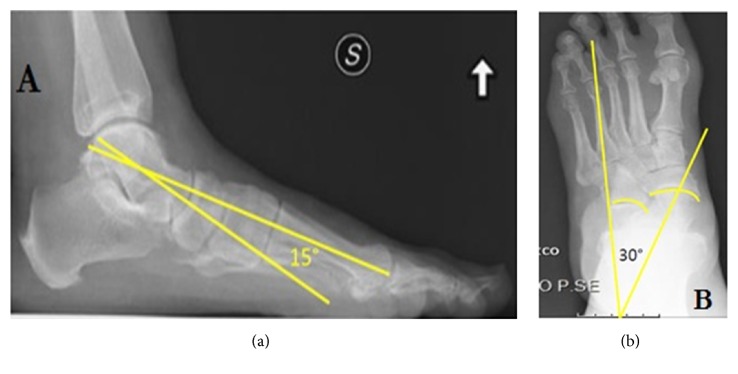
Preoperative radiographic evaluation. Meary's Angle (a) and Kite's Angle (b).
